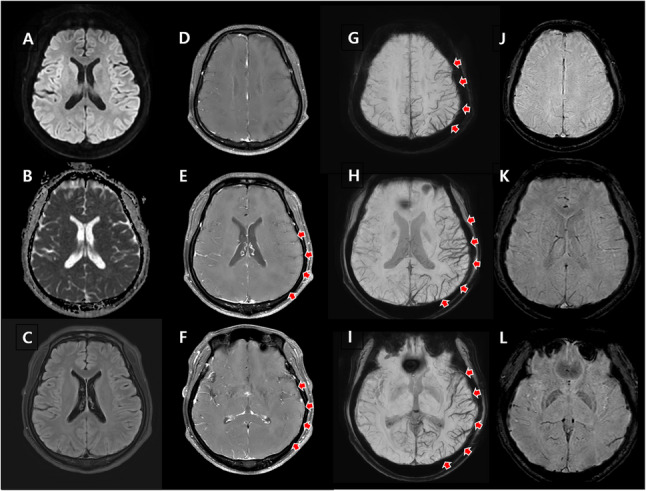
Multimodal brain MRI on the day of headache onset (A–I) and at follow-up (J–L) in a patient with familial hemiplegic migraine type 2 (FHM2). A-C Initial axial DWI, ADC, and FLAIR images (7 h after symptom onset) show no diffusion restriction or parenchymal signal changes. D–F Post-contrast T1-weighted images demonstrate decreased enhancement in the left hemisphere compared to the right. (red arrows). G–I Susceptibility-weighted imaging (SWI; GE SWAN, MinIP reconstruction) at the time of acute presentation reveals prominent cortical veins in the left hemisphere, suggesting asymmetric microvascular hyperemia (red arrows). J–L Follow-up SWI (Siemens, magnitude images) on hospital day 3 demonstrates complete resolution of the previously observed cortical venous prominence
